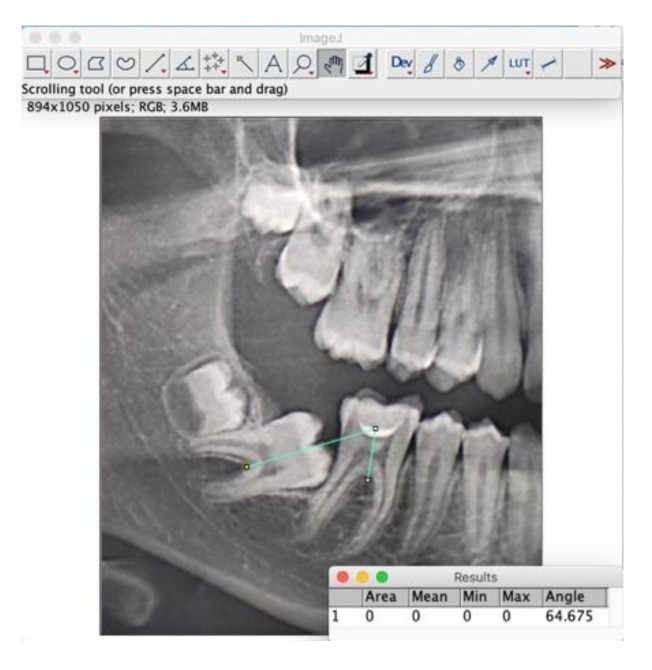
Radiographic measurement performed using ImageJ software.
